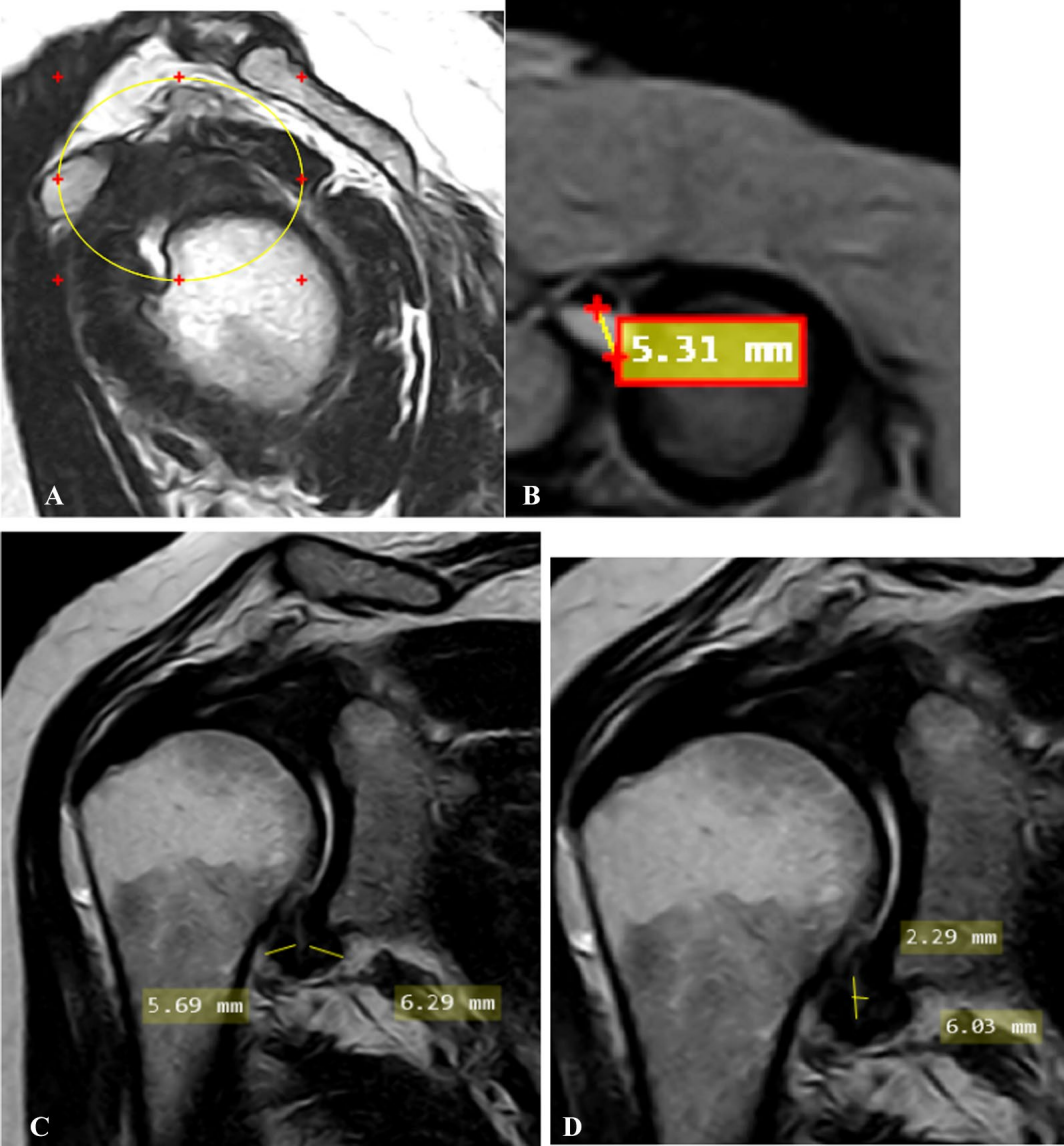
A Oblique sagittal T2-weighted MRI image shows complete obliteration of subcoracoid fat triangle (encircled area). B Oblique axial fat- suppressed PD weighted MRI image shows effusion at long head biceps tendon sheath more than 2mm. C Oblique coronal T2-weighted MR image shows measurement of thickest portion of joint capsule in humeral and glenoid portions of axillary recess. D Oblique coronal T2-weighted MR image shows measurement of maximal height and width of axillary recess
